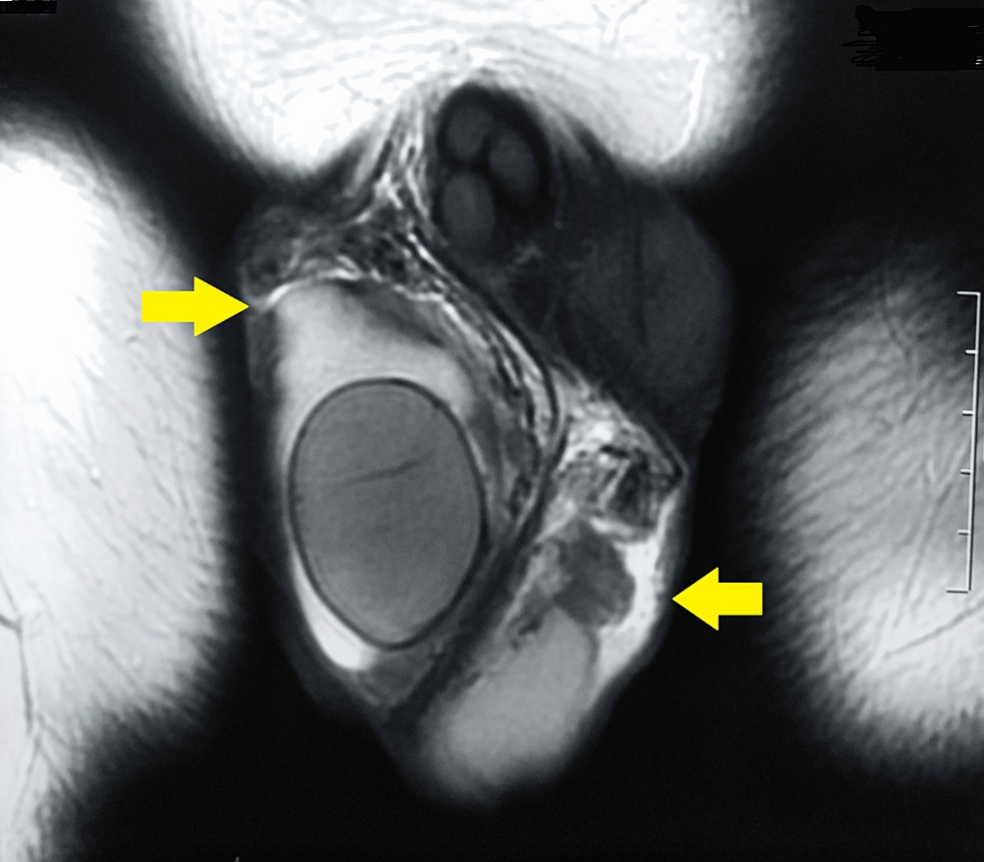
MRI of the scrotum suggestive of bilateral involvement of the epididymis MRI: Magnetic Resonance Imaging
